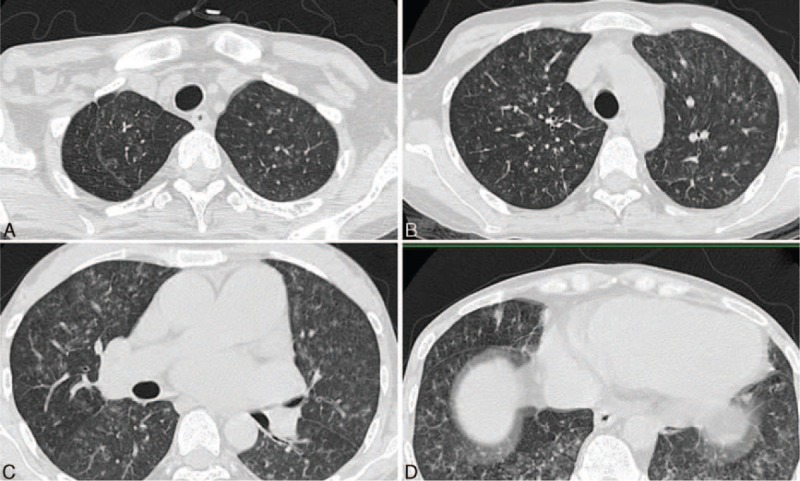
Noncontrast computed tomography (CT) demonstrated widespread miliary opacities in lungs. CT = computed tomography.
